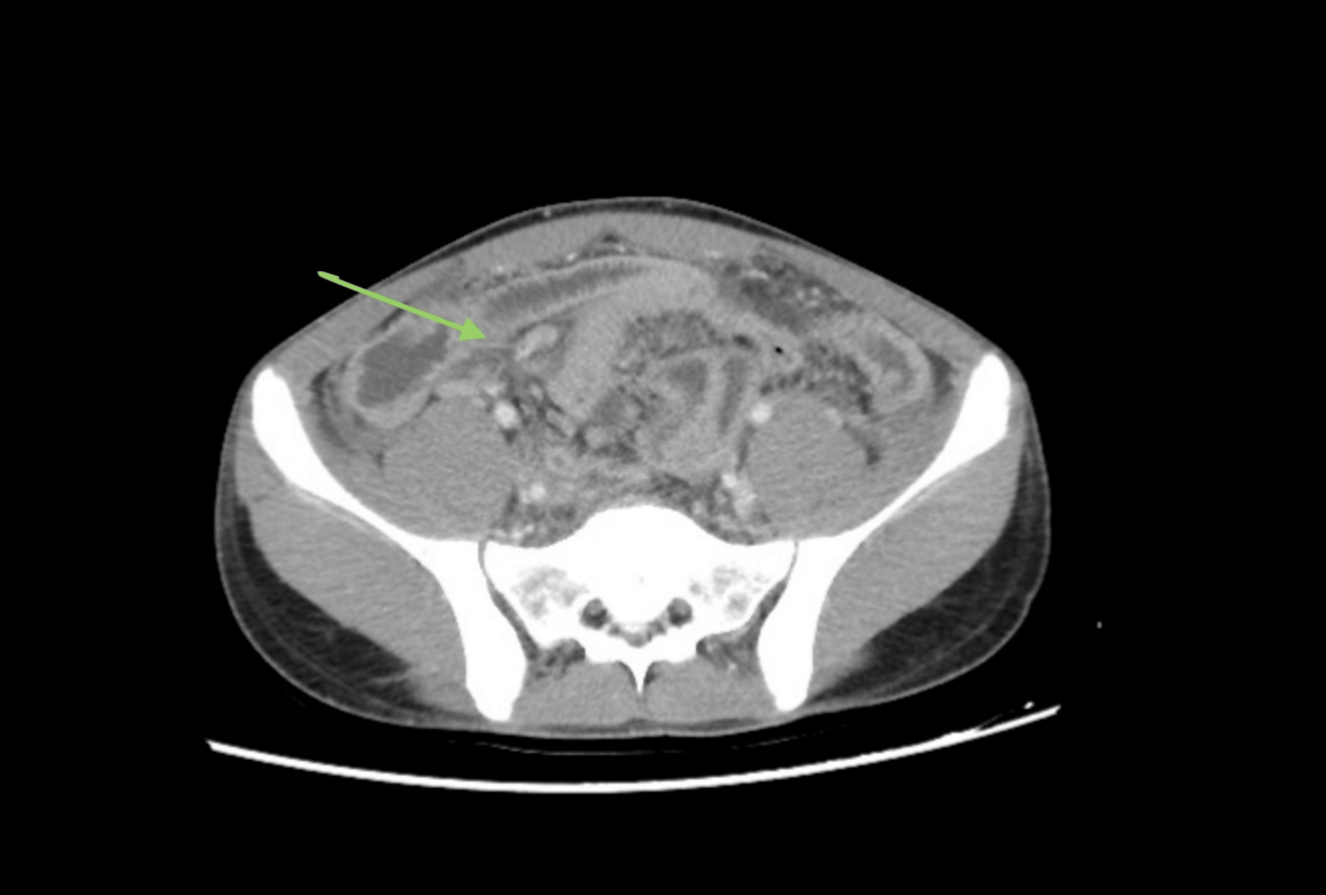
CT image demonstrating a distended, fluid-filled appendix (9 mm in diameter) with surrounding fat stranding—features consistent with acute appendicitis.
